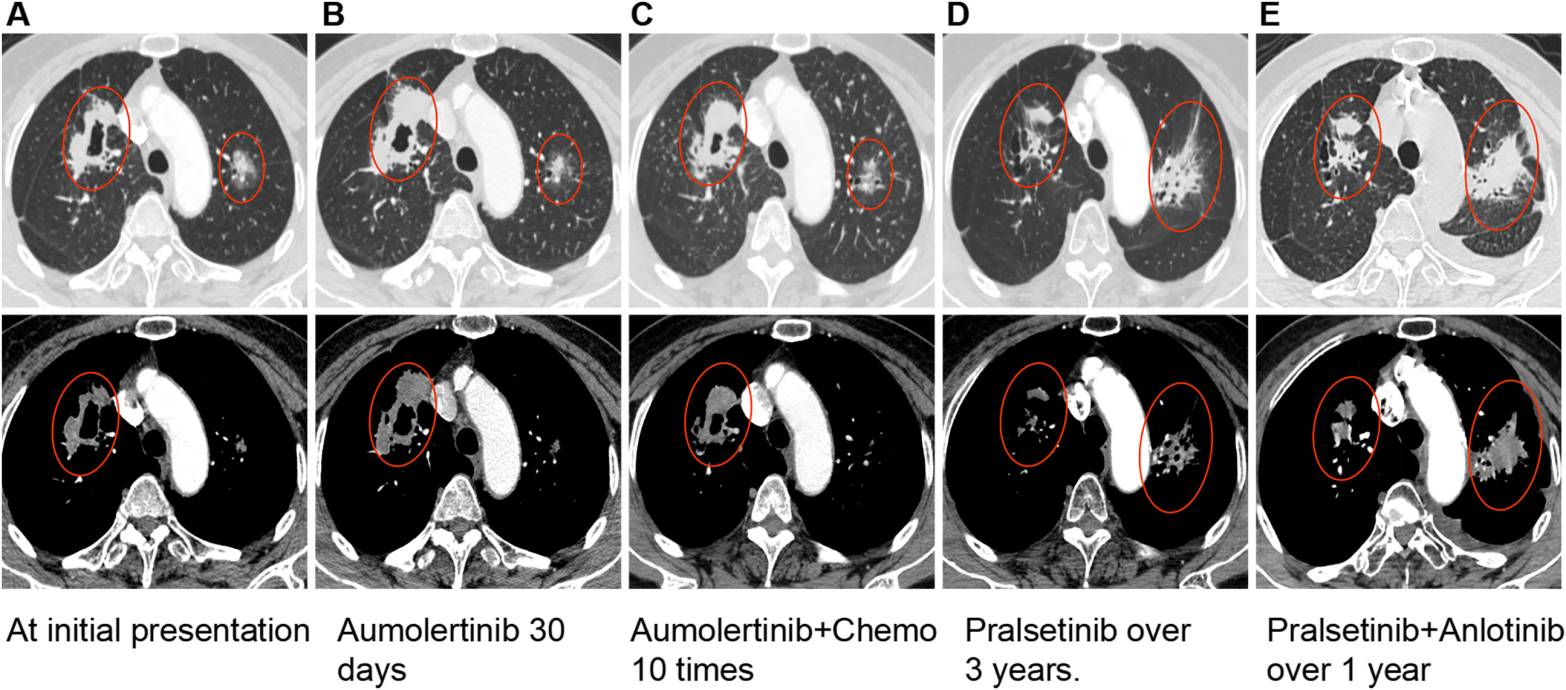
Serial chest CT scans showing the evolution of bilateral lung lesions. (A) At initial diagnosis; (B) One month after initiation of aumolertinib; (C) At approximately 10 months after diagnosis, demonstrating disease progression; (D) At approximately 52 months after diagnosis (nearly 3 years after starting pralsetinib), showing response in the right lung lesion but progression in the left; (E) At approximately 64 months after diagnosis (12 months after initiating pralsetinib plus anlotinib), revealing further enlargement of the left lung lesion with stable disease in the right.
